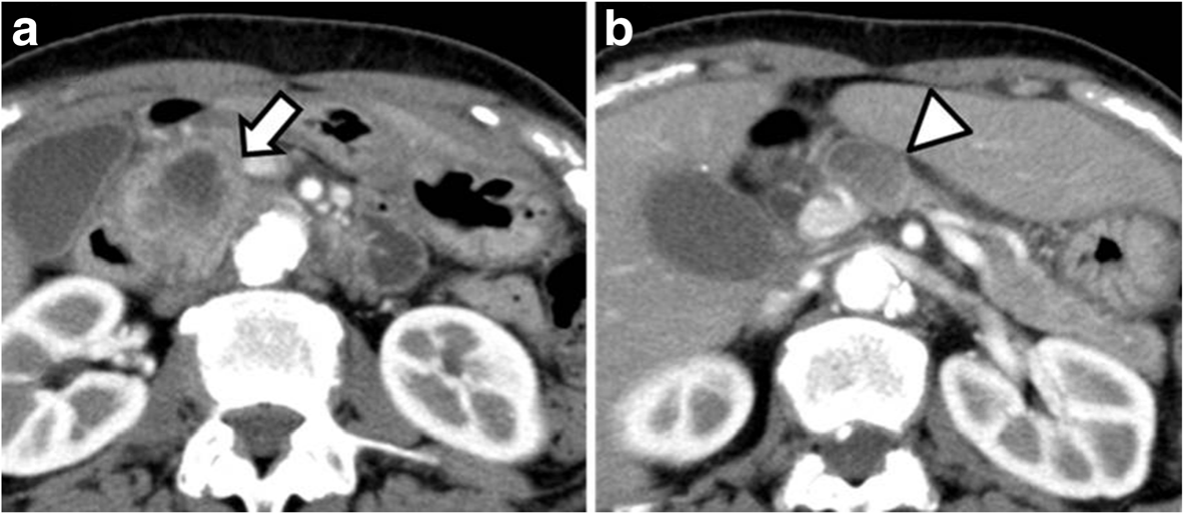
CT showing the primary tumor (a; *arrow*) and a swollen lymph node (b; *arrowhead*)
